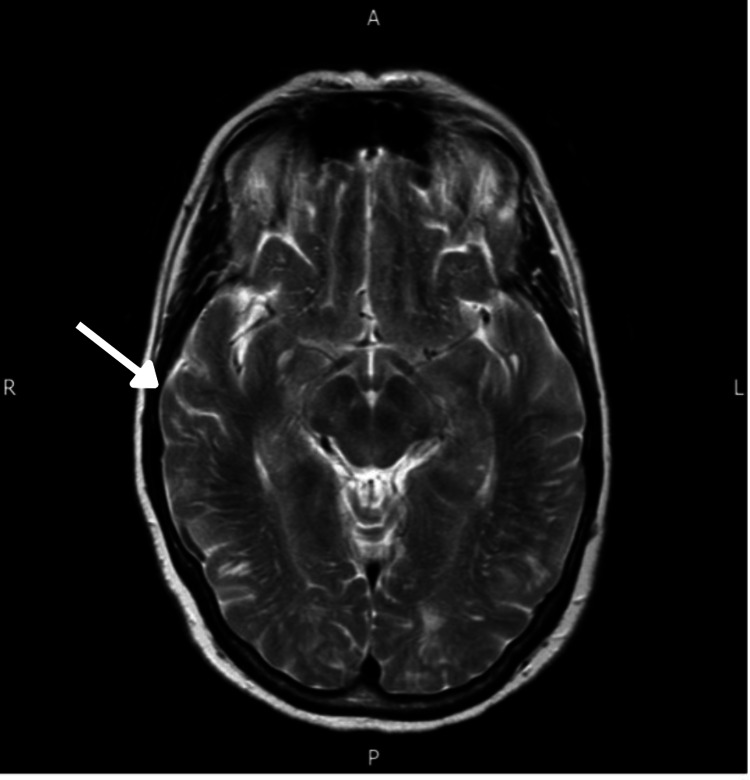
MRI performed in October 2013 showed complete resolution of the hyperintensities (arrow). MRI, magnetic resonance imaging
